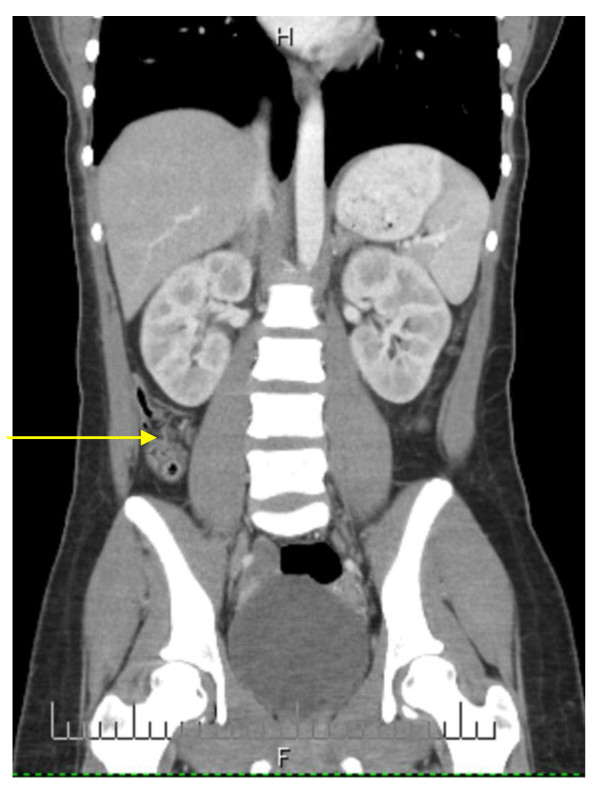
Coronal computed tomography images depicting the appendiceal duplication (arrows).
